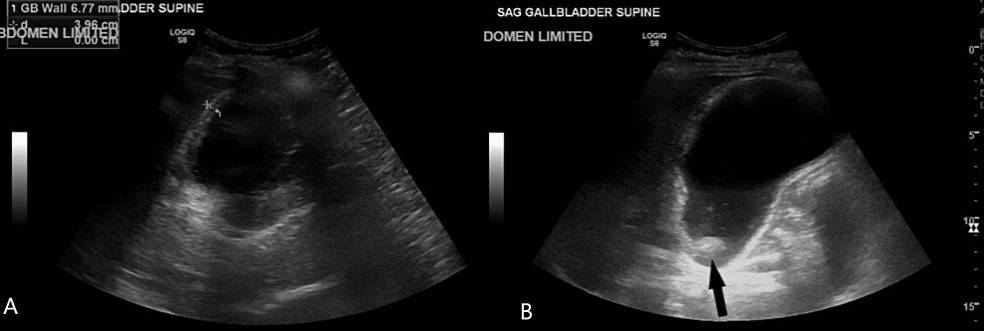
Ultrasound of the abdomen revealing cholelithiasis and acute cholecystitis There is no intra or extrahepatic biliary ductal dilation and the common duct is normal in caliber measuring 4.5 mm. The gallbladder is distended with stones (black arrow, B) and wall thickening measuring up to 6.8 mm (measurement, A). Both images (A & B) are of the gallbladder in the supine position. There is trace pericholecystic fluid and a positive sonographic Murphy's sign.
